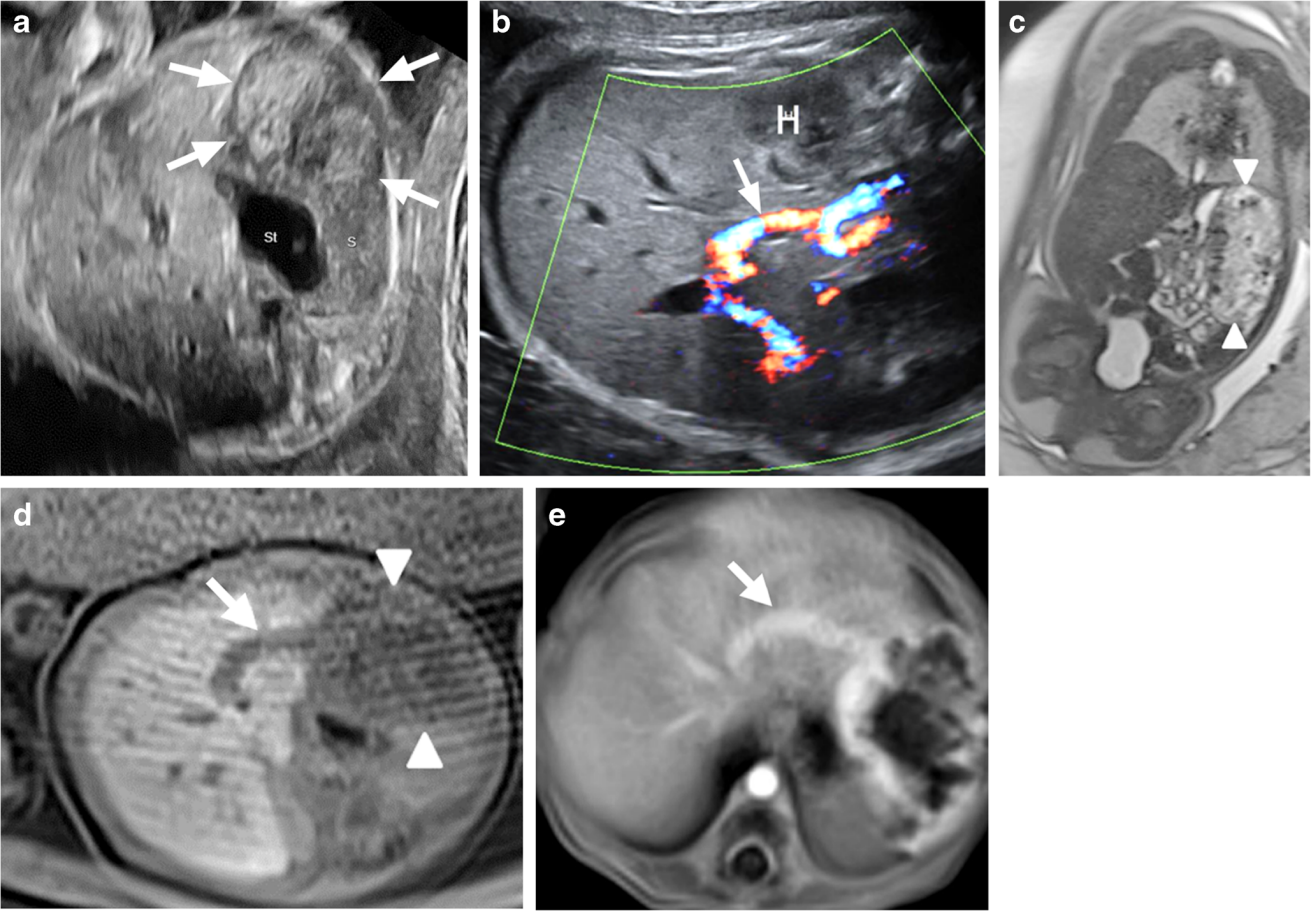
A male fetus at 33 weeks’ gestation (N22) with hepatic hemangioma and postnatal images at 4 days old. a Transverse US image shows a voluminous, solid appearing, heterogeneous left hepatic mass. *Arrows* indicate the borders of the lesion. *S* spleen, *St* stomach. b–d Axial Doppler US image (b), coronal T2-weighted (c) (repetition time [TR]/echo time [TE] 1,000/92 ms) image at the same level as well as axial T2-weighted (d) MR image (TR/TE 6.60/3.30 ms) show the inhomogeneous, exophytic growing lesion arising from the left hepatic lobe (*H* in b, *arrowheads* in c, d). Note the numerous tubular forming flow voids indicating vascular structures (*arrow* in d) and the extreme enlarged left hepatic vein (*arrow* in b). e Postnatal T1-weighted MR image (TR/TE 3.61/1.61 ms) after contrast shows the marked early peripheral enhancement of the lesion at the arterial phase and confirms the enlarged left hepatic vein (*arrow*). After significant regression, the tumor was resected when the boy was 18 months old
